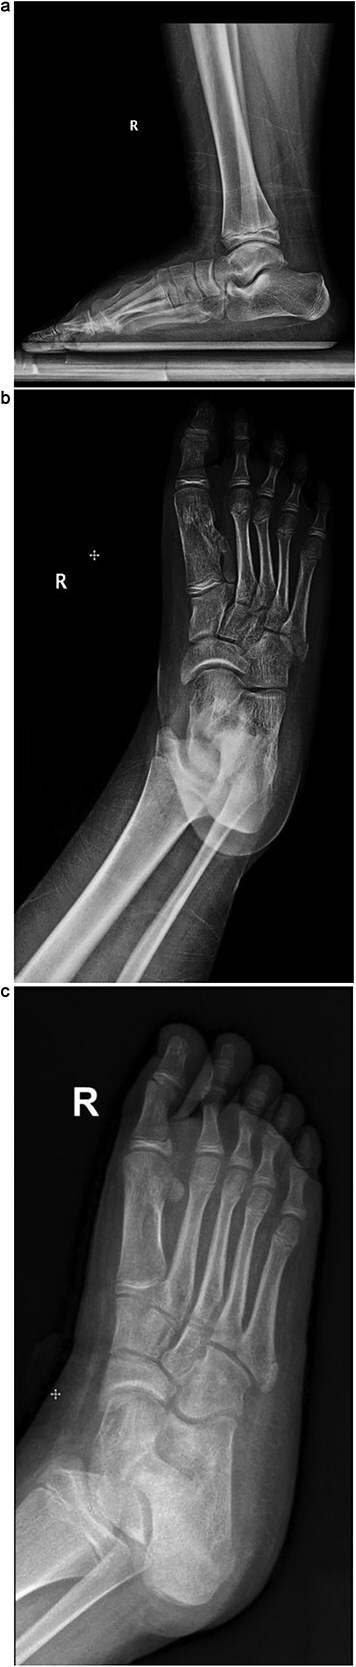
(a) Lateral radiograph of the right foot demonstrating a bony mass extending from the medial cuneiform to the first metatarsal. (b) Oblique radiograph of the right foot highlighting the abnormal bony outgrowth between the first and second metatarsals. (c) Anteroposterior radiograph showing a bony mass arising from the medial cuneiform extending toward the first metatarsal, with widening of the first intermetatarsal space.
